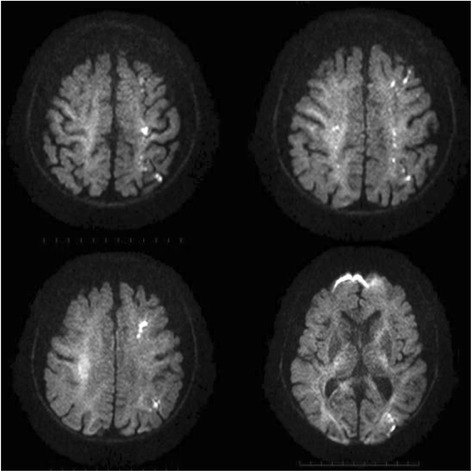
Brain MRI (diffusion-weighted) shows multiple small brain infarctions
